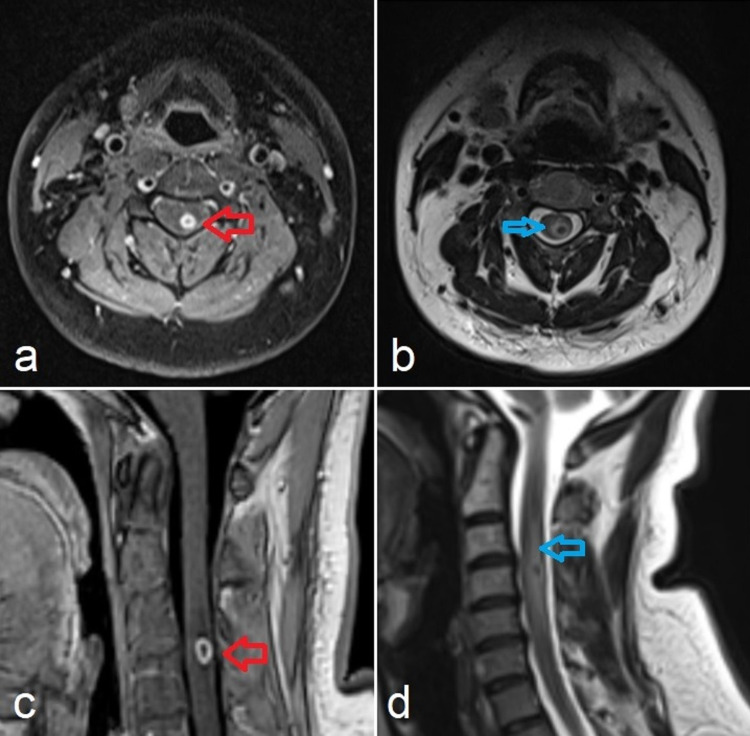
MRI images of the cervical spine, (a) axial and (c) sagittal T1WI post-contrast sequences, shows a ring-enhancing lesion (red arrows) in spinal cord opposite C4 vertebral body with surrounding peripheral edema, blue arrows in T2WI axial (b) and sagittal (d). MRI, magnetic resonance imaging; T1WI, T1-weighted image; T2WI, T2-weighted image
